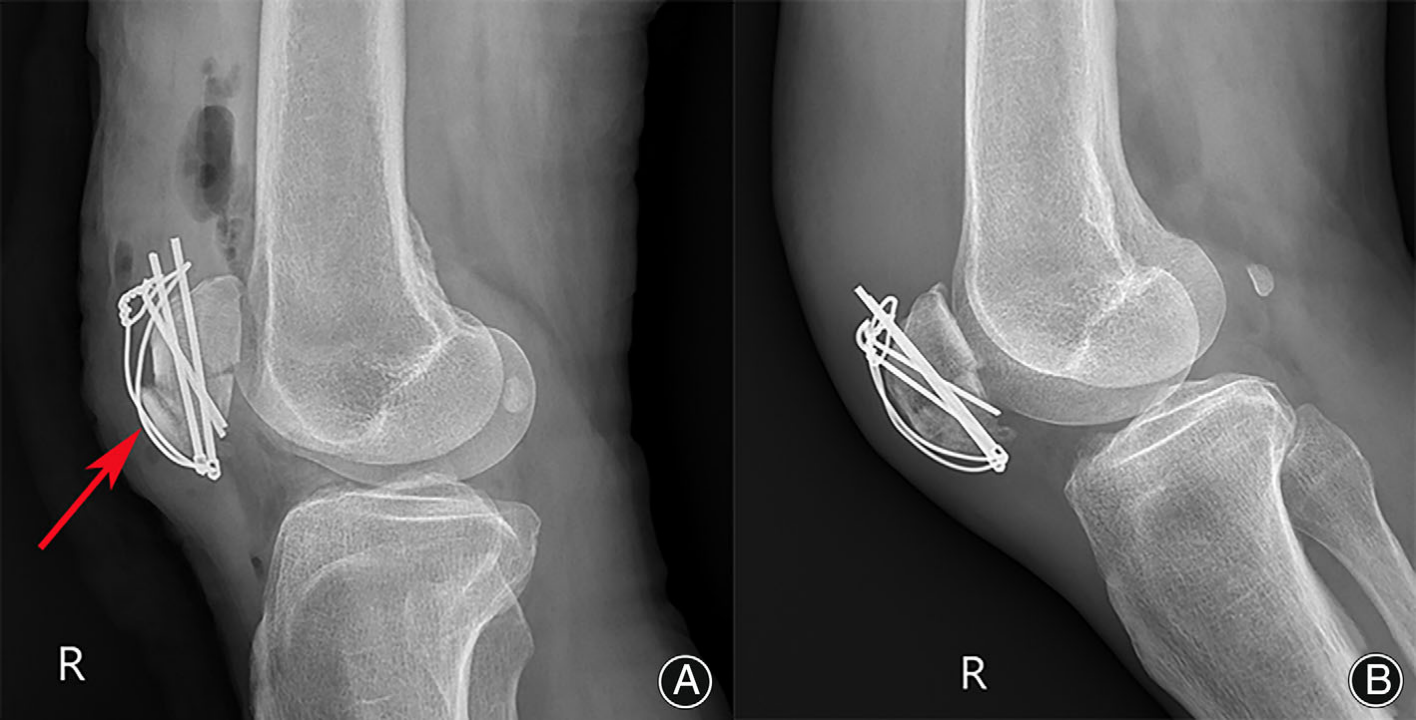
Postoperative lateral radiographs of the knee showing anterior displacement of the fracture fragment at the inferior pole of the patella (red arrow) (A). Lateral radiographs showing blurring of the fracture line and anatomical reduction of the anteriorly displaced fracture fragment at 1 month postoperatively (B).
